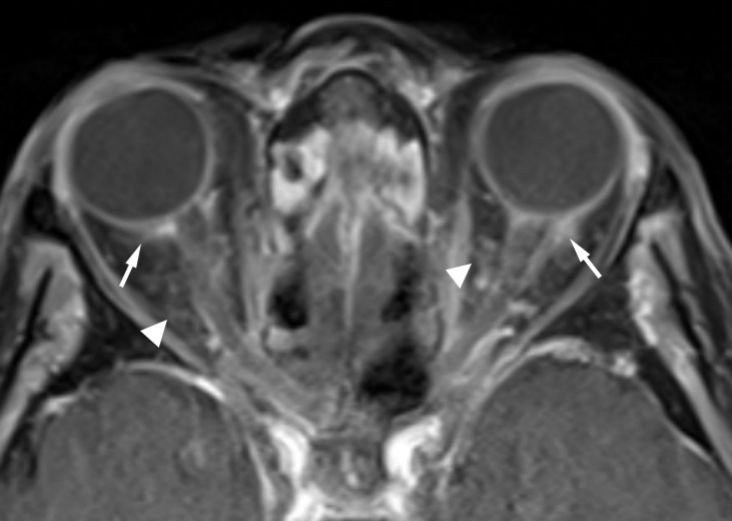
Post-Gd axial T1-weighted MR image with fat saturation shows enhancement of bilateral posterior sclera extending to the adjacent optic nerve sheath (arrows); findings are consistent with scleritis. Also noted is mild ill-defined enhancement in retro-conal fat (arrowheads), consistent with mild post-septal cellulitis.
